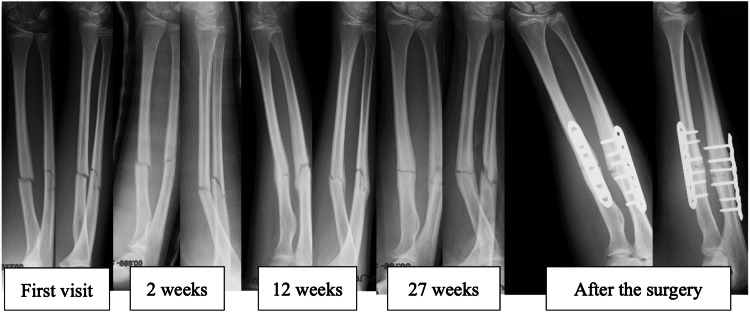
The secondary 14-year-old male X-ray observations were made at the initial visit, 2 weeks, 12 weeks, 27 weeks, and post-surgery in the same week. The minor dislocation was noted initially, with a slight angular deformity developing by the 2-week mark. No further deformity progression was observed, and bone fusion was achieved at 12 weeks. However, a re-fracture occurred at 27 weeks, leading to the performance of a double-plate procedure.
